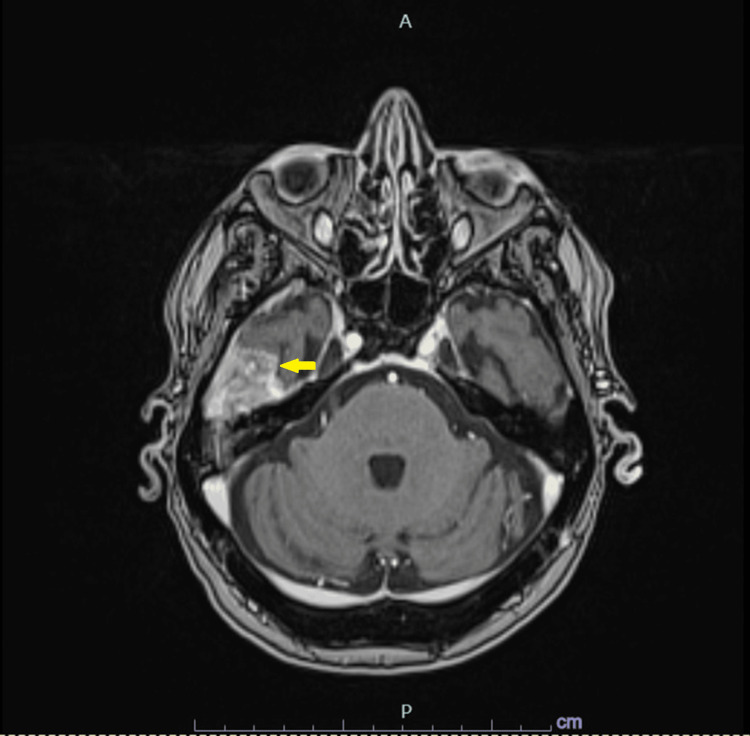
MRI of the brain showcasing the lesion with involvement of the middle fossa.
